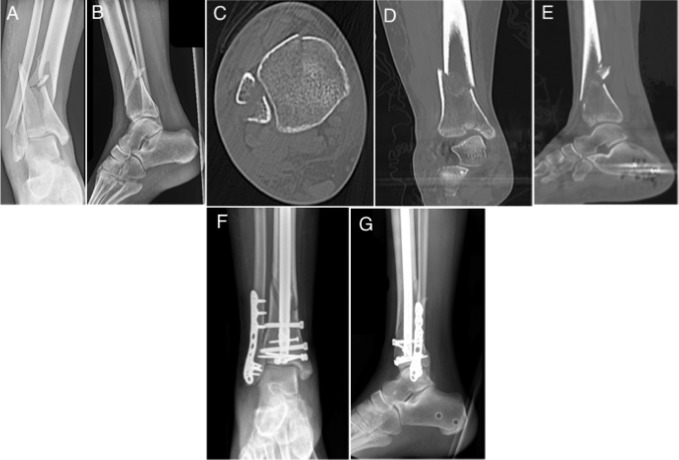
Radiograph demonstrating the (A) AP and (B) lateral injuries and (C–E) injury CT images of an intra-articular distal tibial fracture. Postoperative (F) AP and (G) lateral radiographs after intramedullary nail and screw fixation of the articular fracture.
